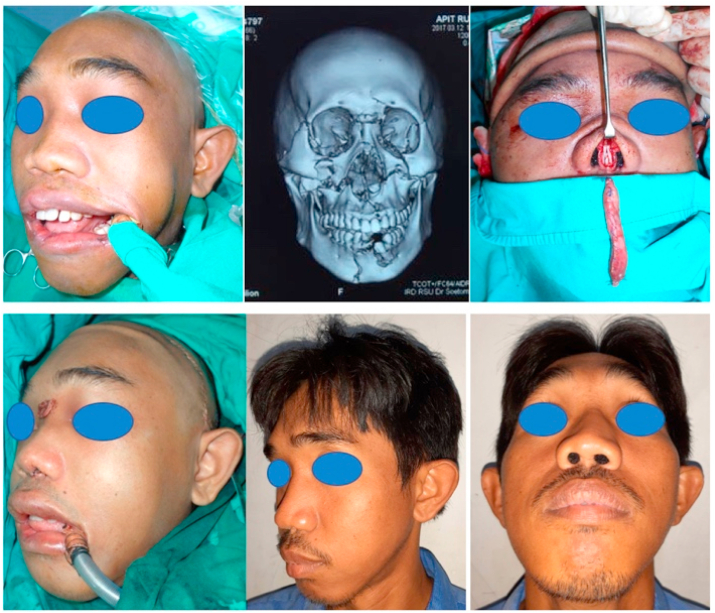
Above (Left to Right) Pre-op oblique view of the patient; Pre-op three-dimension CT scan of the patient; Pre-insertion of the diced cartilage fascia graft. Below (Left to right) Post-op oblique lateral view of the patient; Four years post-op oblique view of the patient; Four years post-op malar view of the patient.
